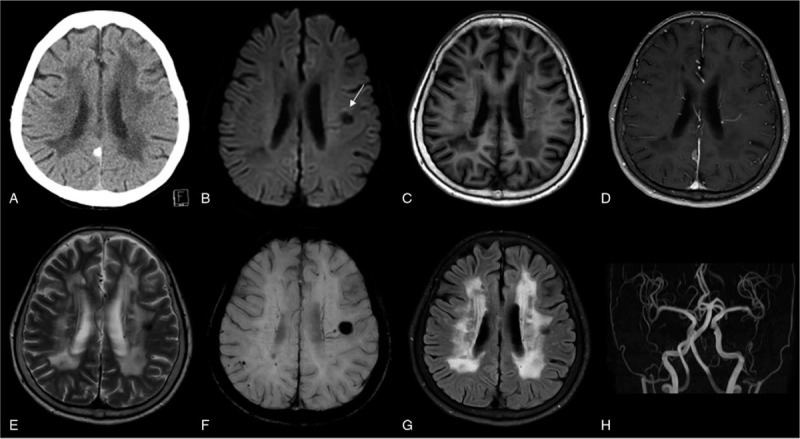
Brain computed tomography image obtained on the day of admission does not show any acute hemorrhage (A). Brain magnetic resonance image with contrast enhancement obtained 3 hours after the initial CT. The diffusion-weighted image shows decreased signal intensity in the left corona radiata and a perilesional high signal intensity towards the motor cortex (arrow) (B). T1 weighted image shows isointensity without enhancement (C, D). T2 weighted image (E) and Susceptibility-weighted imaging (F) show low signal intensity compatible with acute hemorrhage. The subcortical white matter changes seen in fluid-attenuated inversion recovery images were unchanged from the previous images (G). Brain magnetic resonance angiography shows no significant stenotic lesions in intracranial arteries (H).
